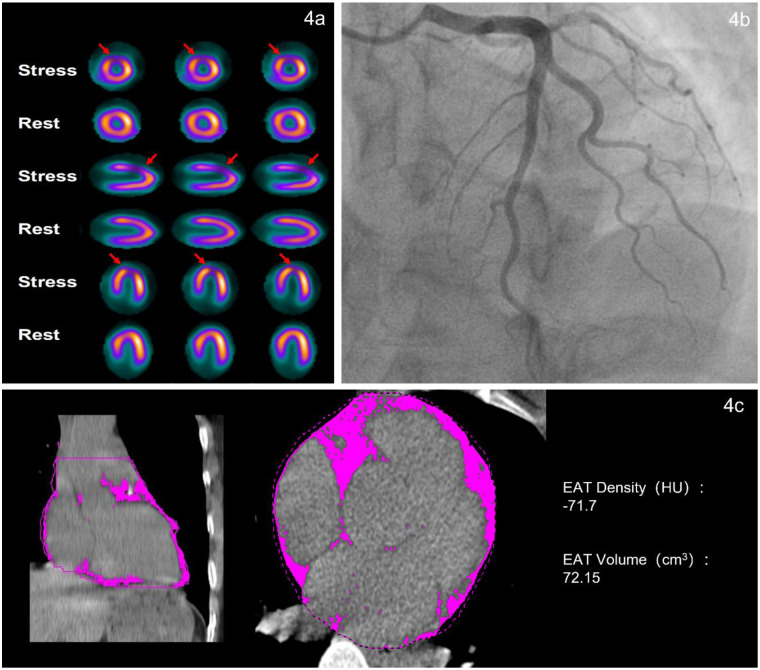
Typical case of patient with INOCA. The patient is a 43-year-old female admitted to the hospital due to chest tightness for 2 months. The EAT density is −71.7 HU. Blood test indicators showed CRP 0.98 mg/L, BNP 92 ng/L, and cTnI0.0021 ng/mL. (a) Stress-rest myocardial perfusion imaging revealed reversible myocardial ischemia in the partial anterior wall, apex, and anterior septal wall of the left ventricle. (b) CAG revealed no significant stenosis (<50% luminal narrowing) in LAD, LCX or RCA. (c) Non-contrast CT scan shows cross-sectional and coronal views of epicardial adipose tissue (the pink area).
